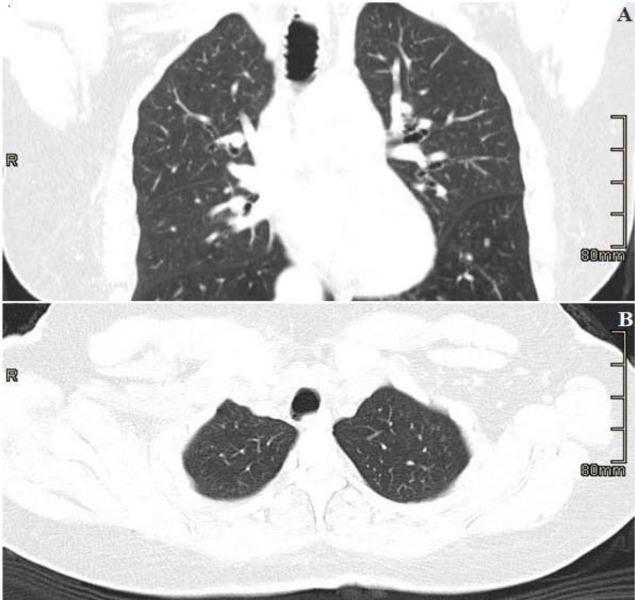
A) HRCT scan images showing prominent trachea; B) posterior diverticuli
